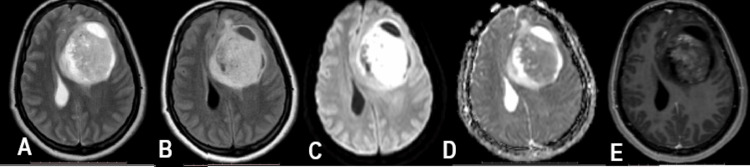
MRI brain, axial slices; T2 Weighted Image (T2WI), Fluid Attenuation Inversion Recovery (FLAIR), Diffusion Weighted Image (DWI), Apparent Diffusion Coefficient (ADC) and Contrast Enhanced T1 Images (C+T1WI), sequences. Predominantly solid intra-axial, supratentorial lesion in the left frontal lobe causing mass effect in the form of midline shift and compression upon the ipsilateral lateral ventricle. It is returning hyperintense T2 and FLAIR signals (A & B), demonstrating diffusion restriction on DWI and ADC (C & D) and post-contrast enhancement (E)
